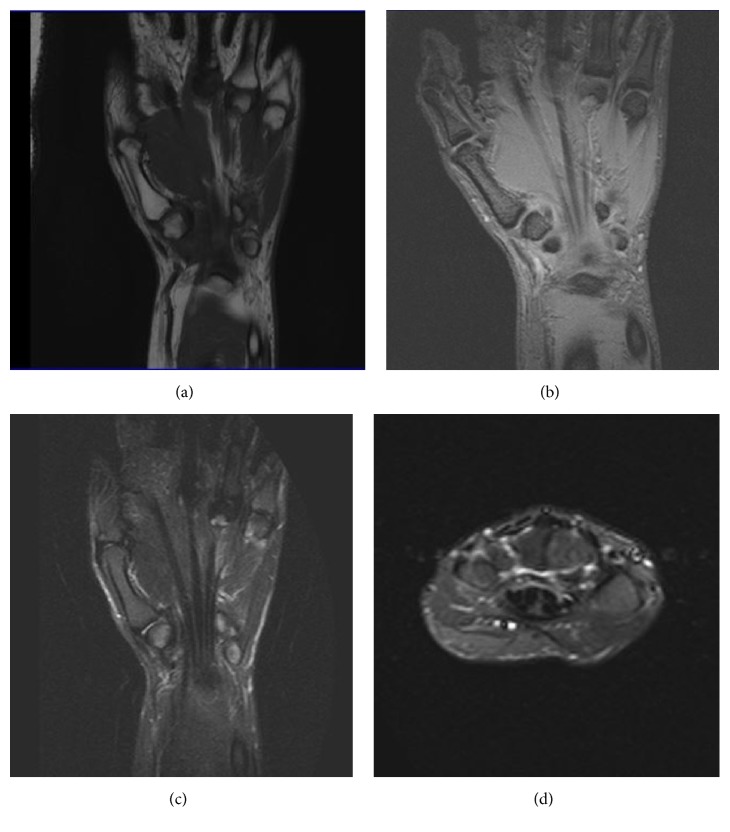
After three months, an MRI scan reveals an almost normal trapezium with patient's symptoms fully subsided. (a) TIWI coronal image. (b) PD coronal image. ((c) and (d)) STIR coronal and axial images.
